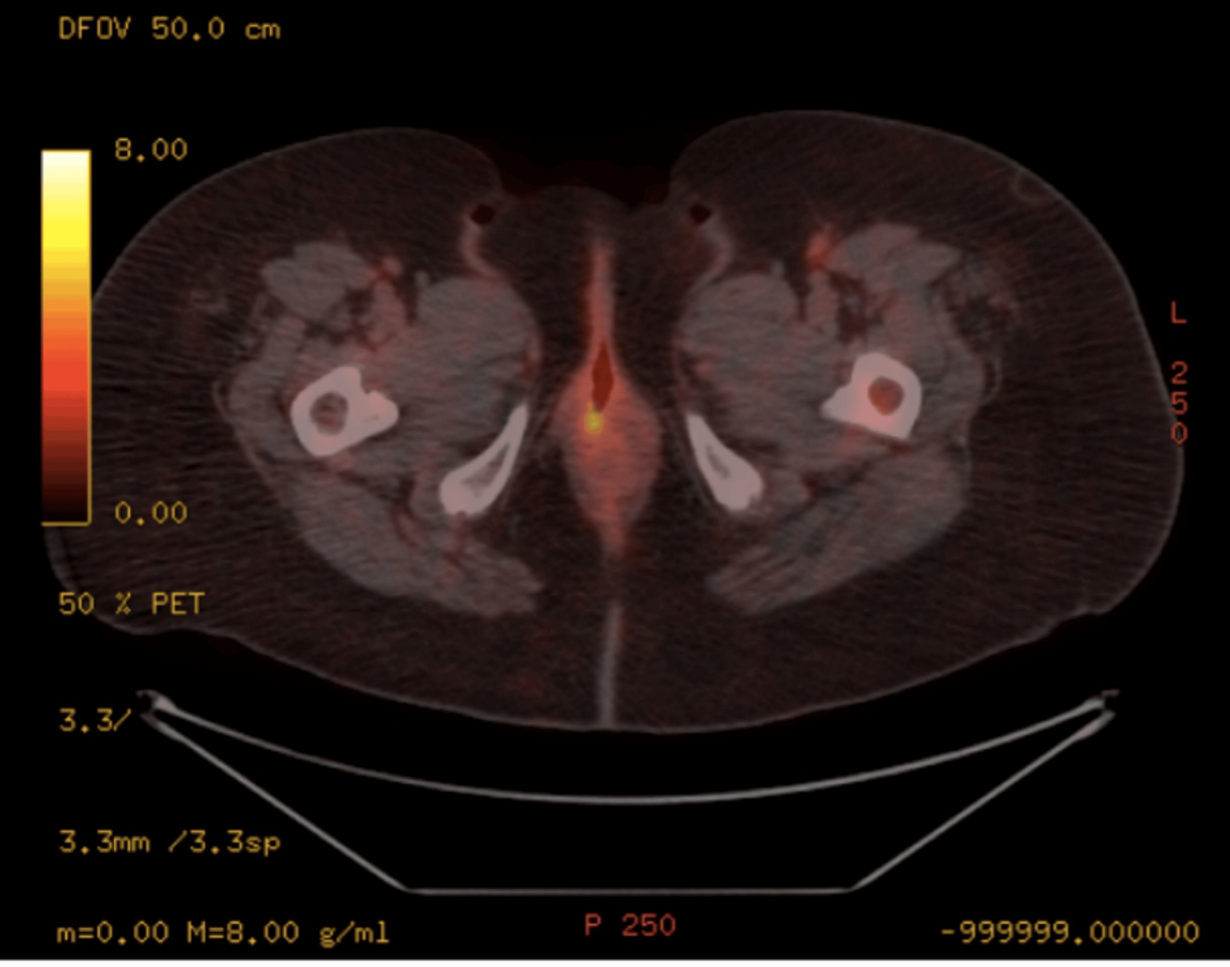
FDG and gallium-68 DOTATATE PET-CT demonstrating a small area of FDG accumulation in the right posterior wall of the vagina DFOV, dosimetric field of view; FDG, fluorodeoxyglucose; PET-CT, positron emission tomography-computed tomography
